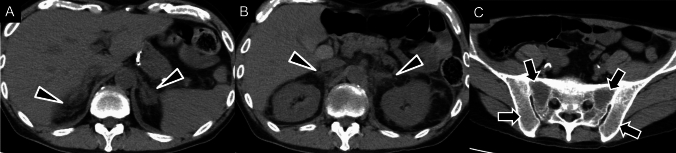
Adrenal Infarction in a patient with myelodysplastic syndrome. A male in his 50 s presenting with acute onset of abdominal pain. A, B Unenhanced computed tomography (CT) images showing swelling of the bilateral adrenal glands and increased surrounding fat density (arrowheads), which are consistent with an adrenal infarction. C CT image revealing elevated density in the pelvis and bilateral iliac bones (arrows), probably associated with atypical cells from myelodysplastic syndrome.
